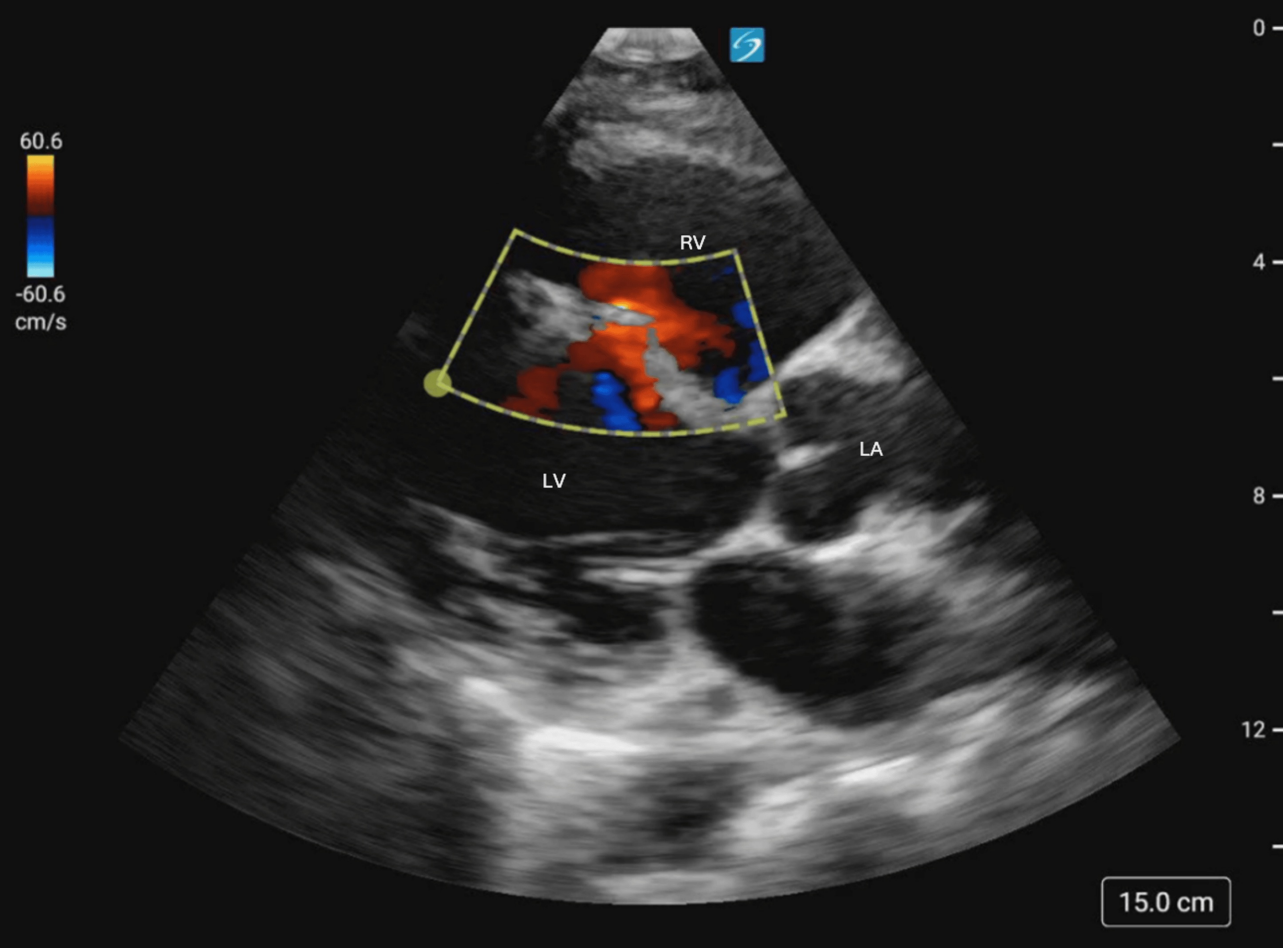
Parasternal long axis view with color doppler with turbulent left to right flow across the ventricular septal wall
